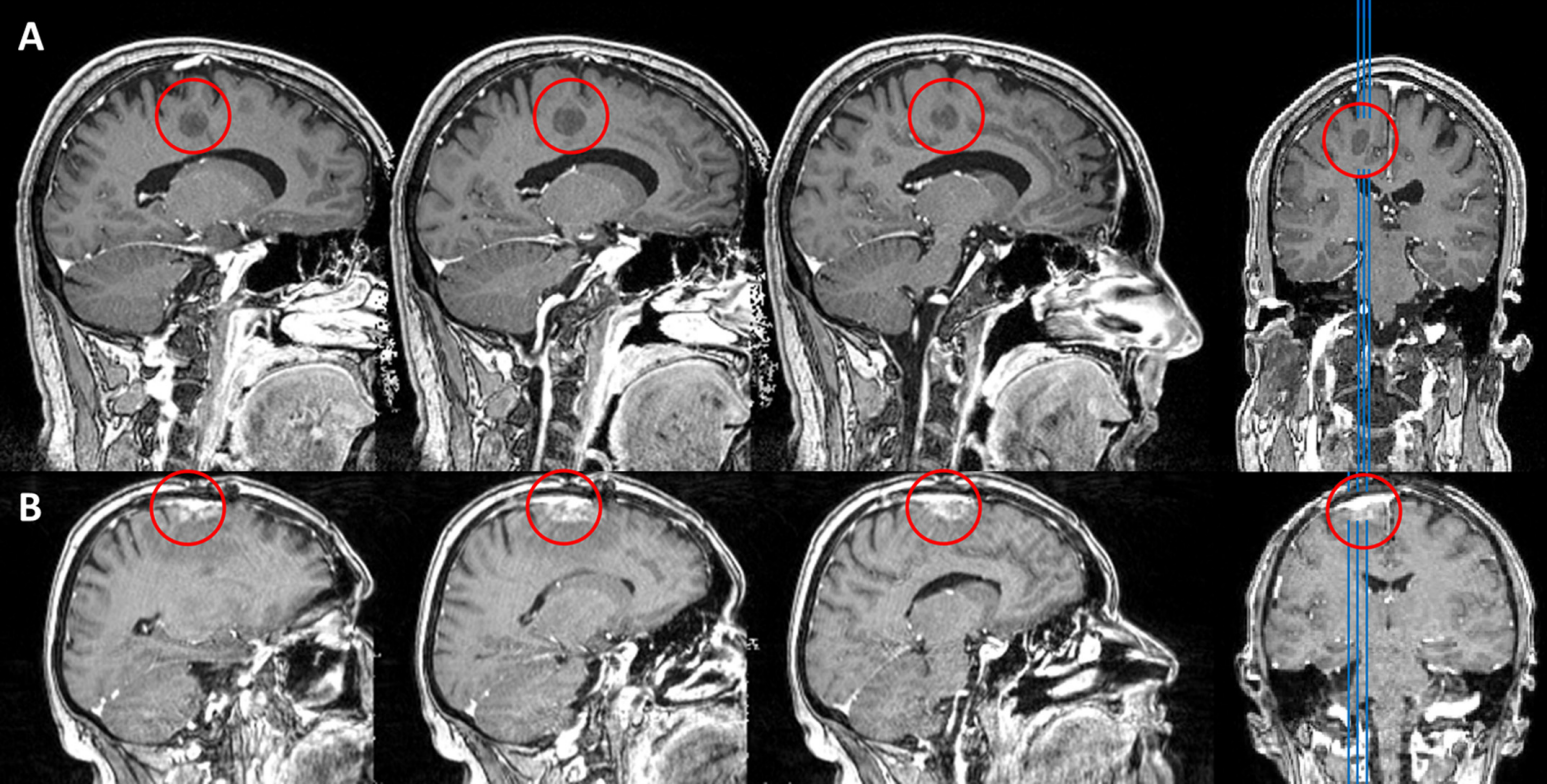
T1 weighted MRI MPRAGE sequences of patients HS (A) and CA (B). Lesion sites are marked with a red circle.
